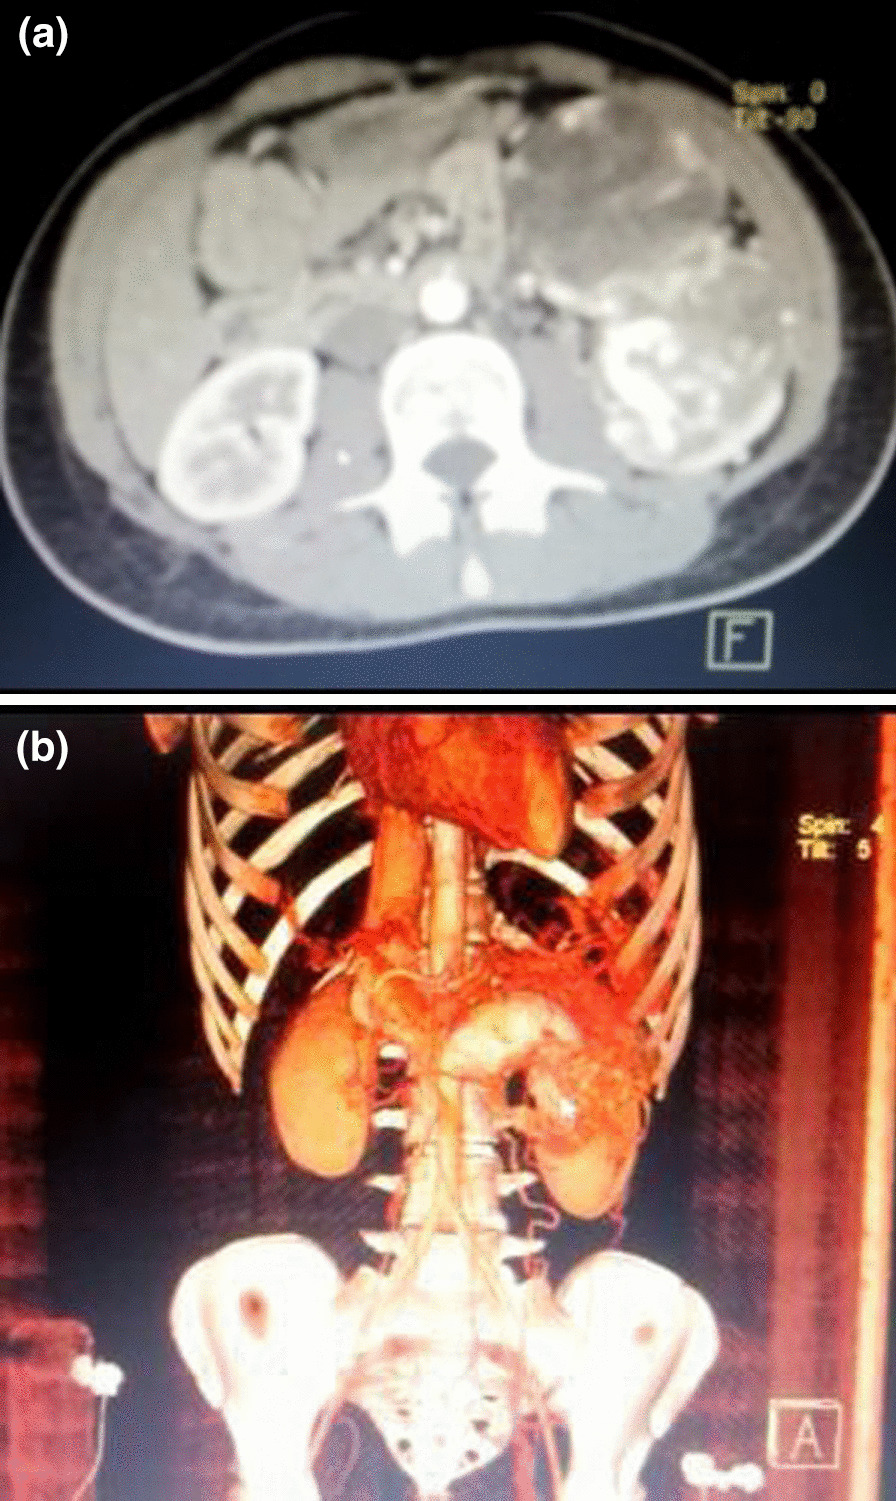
a Computed tomography (CT) scan of the abdomen showing a 7 × 5 × 3 cm^3^ mass arising from the anterior part of the left kidney with tumor thrombus in the inferior vena cava extending to the right atrium without metastases. b CT scan of the abdomen with reconstruction
